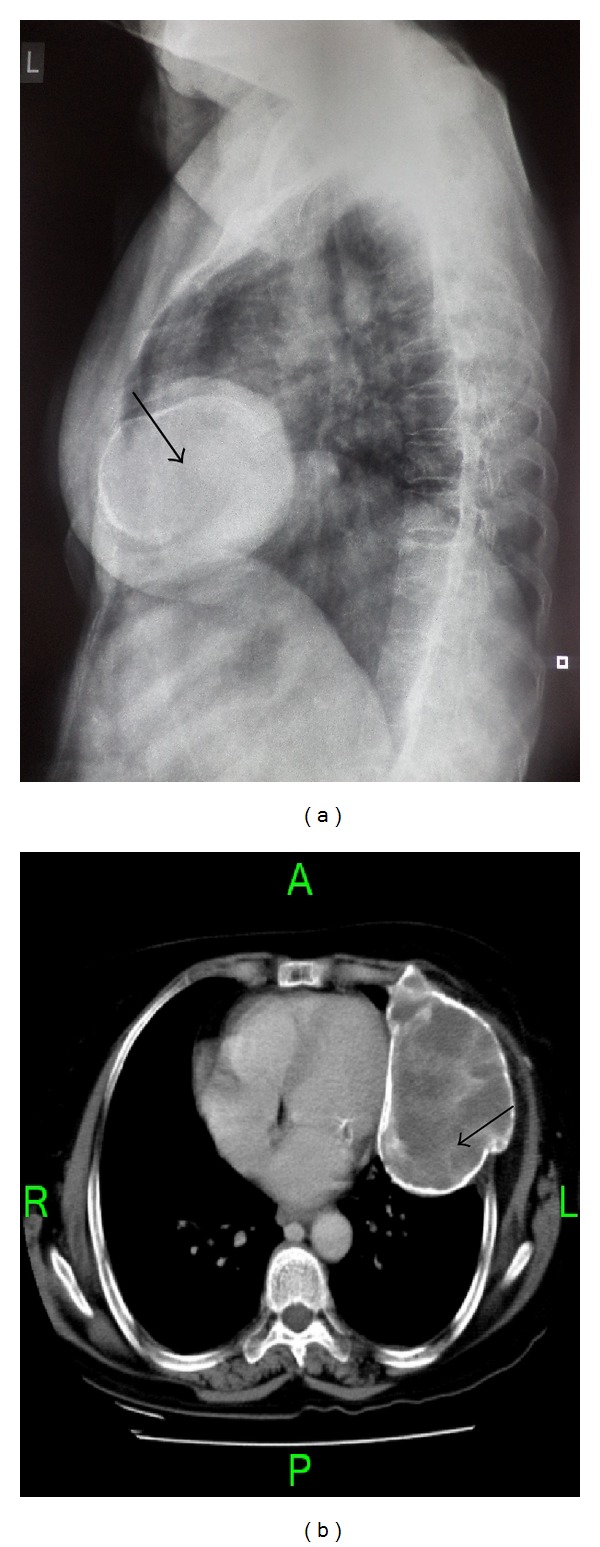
(a) X-ray and (b) CT scan-lobulated, expansile, intramedullary lesion (arrow) with ground-glass centre and thinning of the cortex arising from the anterolateral aspect of the left 5th rib.
